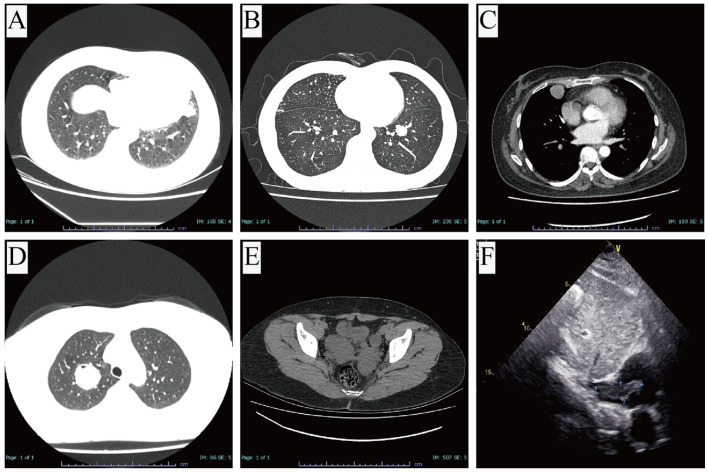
Imaging findings: (A–D) chest computed tomography images of Case 1, Case 4, Case 5, and Case 7, respectively, showing pulmonary nodules in different locations; (E,F) Case 1’s pelvic computed tomography and transthoracic echocardiogram three years after surgery.
